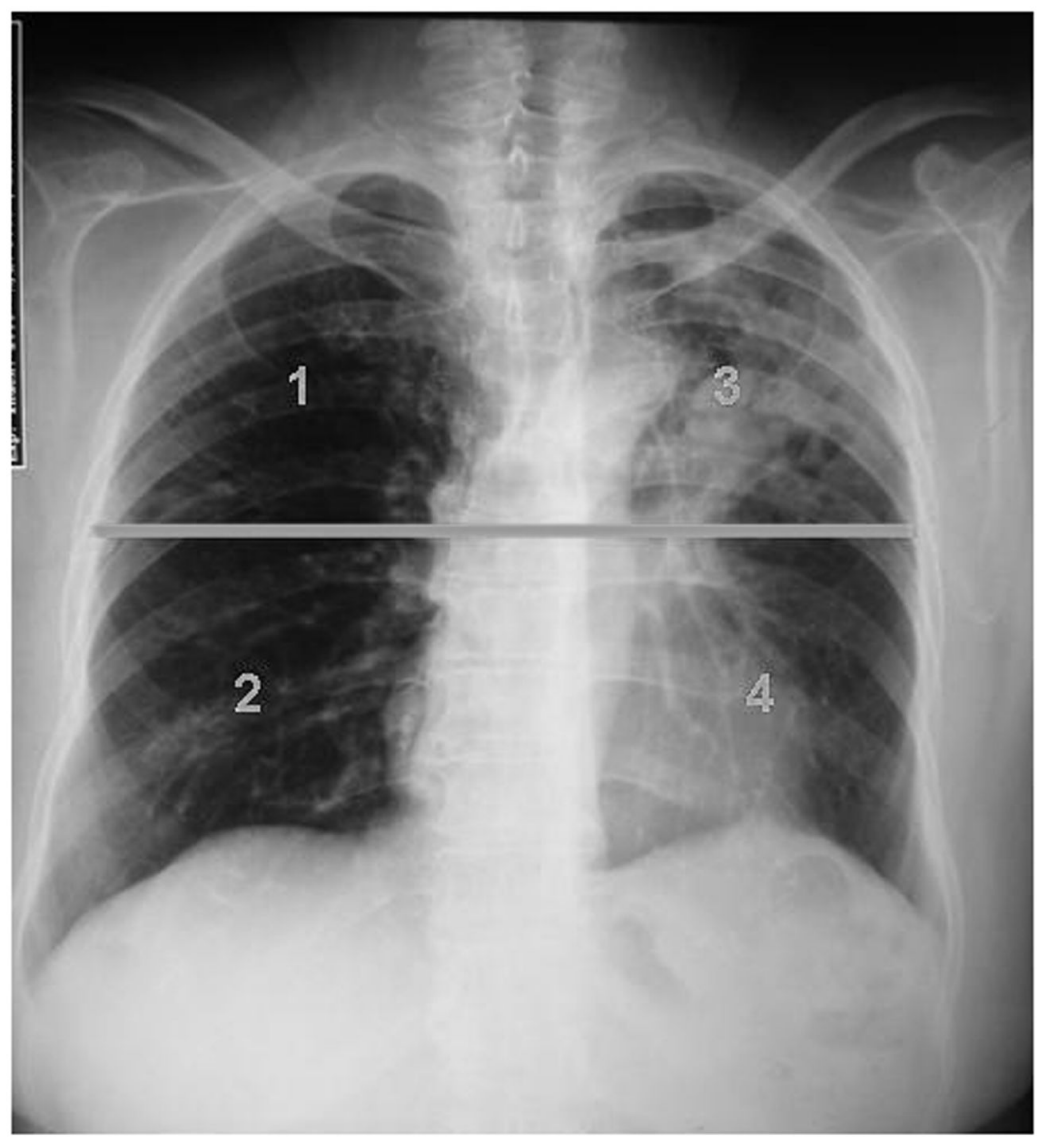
Representative example of the result of the scoring system for radiographic abnormalities. Score =6.5, which represents the mean value of two evaluations.
